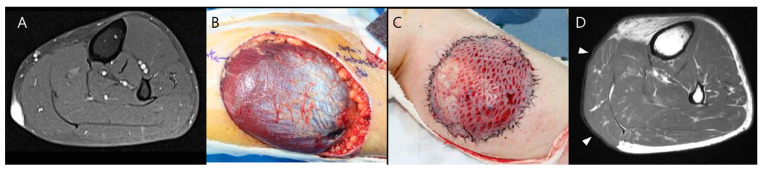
Wide excision for dermatofibrosarcoma protuberans on the calf area. (A) Preoperative axial T1-weighted enhanced MR image shows a well-enhancing soft tissue tumor confined to the skin and subcutaneous fat layer. (B) Tumor bed excision was performed due to the patient’s previous unplanned excision at another hospital, where initial management was undertaken. (C) To address the resultant defect, a split-thickness skin graft was applied to ensure adequate coverage. (D) Postoperative axial T1-weighted MR image after 1 year shows successful skin coverage at the operative site (arrowheads).
